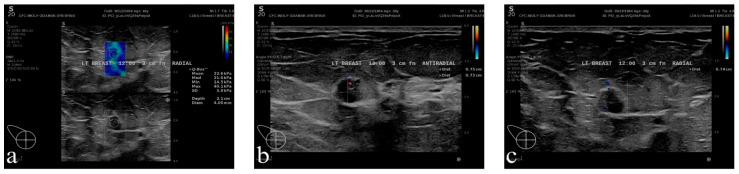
A 40-year-old female patient’s ultrasound pictures presenting to Group C; (a) elastography presentation of palpable area in the left breast (soft lesion), (b) B-mode and microflow imaging presentation of the identified lesion, anti-radial probe setting, (c) B-mode and microflow imaging presentation of the identified lesion, radial probe setting.
